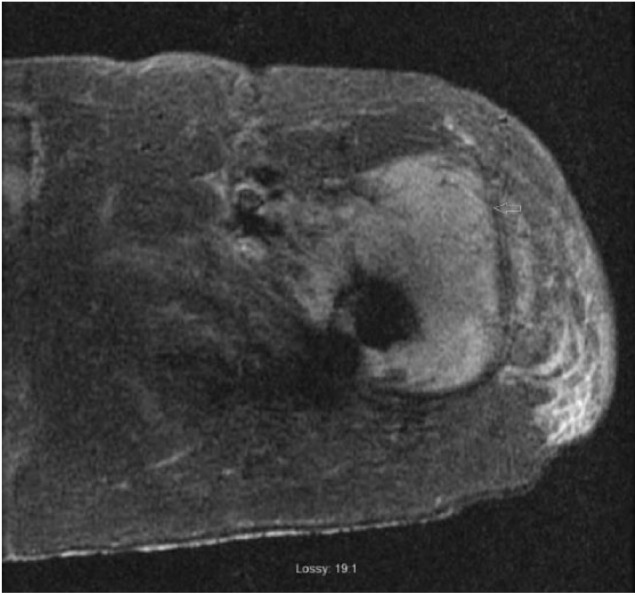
MRI of the hip region with metal artifact reduction protocol. A coronal STIR
